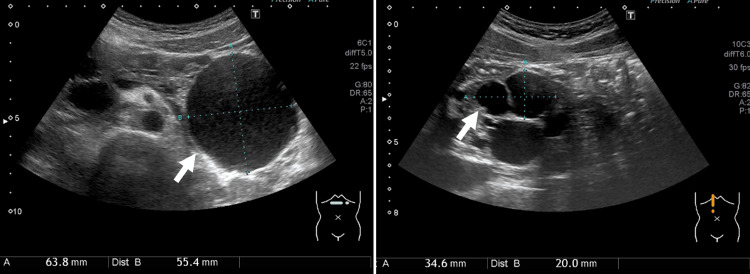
Abdominal ultrasonography performed two months before pregnancy Multiple cystic lesions are seen in the pancreas on abdominal ultrasonography.
